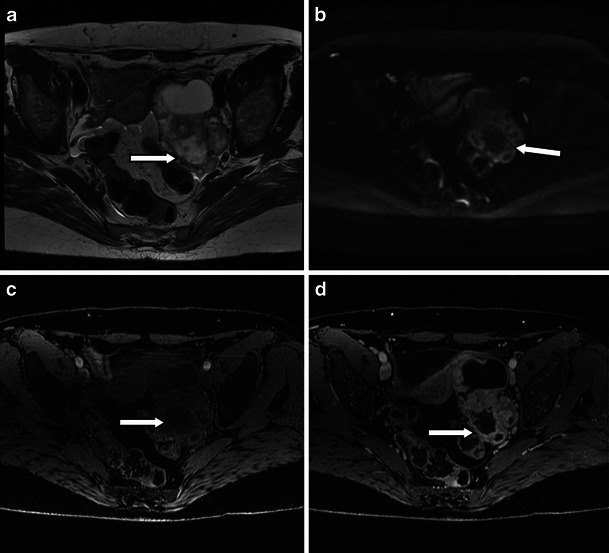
Three-tesla MR images in a 61-year-old patient (same as in Fig. 2) with confirmed benign struma ovarii of the left ovary. An 8-cm left oval-shaped complex multiloculate lesion is seen on the T2-weighted (a), diffusion-weighted images with *b* = 1,200 mm/s^2^ (b) and T1 fat-saturated images (LAVA) pre-gadolinium (c) and post-gadolinium (d) within the left hemi-pelvis. The solid portion of the mixed solid and cystic lesion presents with the typical low signal intensity on the T2-weighted image (a) and intermediate signal intensity on the T1-weighted image (c) (*arrows*). d These solid components enhance markedly (*arrow*) which together with the multilobulate surface resemble a “lacy” pattern. b This lacy pattern in keeping with solid thyroid tissue is also obvious on the diffusion-weighted images (*arrow*)
